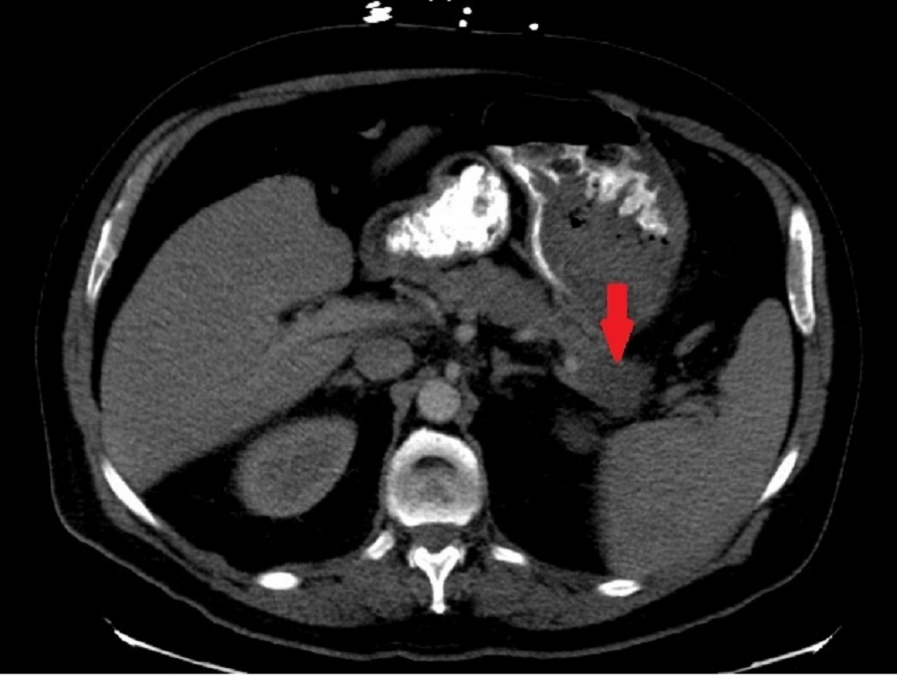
CT scan of the abdomen CT scan of the abdomen showing heterogenous mass (red arrow) within the tail of the pancreas.
